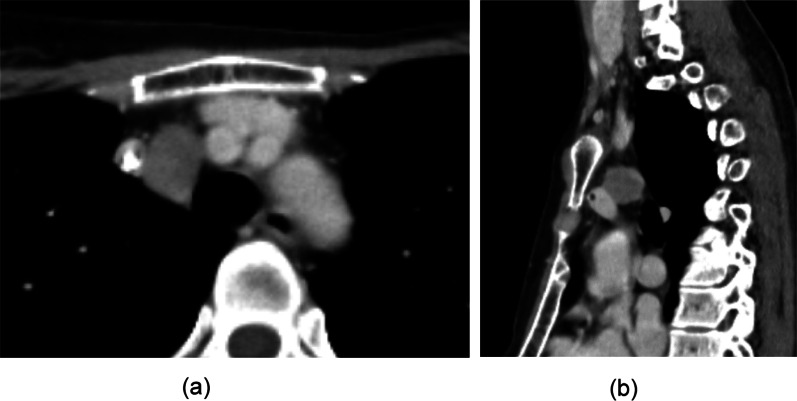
A chest CT scan obtained at 4 years after the patient's breast surgery. A mediastinal nodule (diameter: 23 mm) was detected on the horizontal (a) and sagittal (b) views
